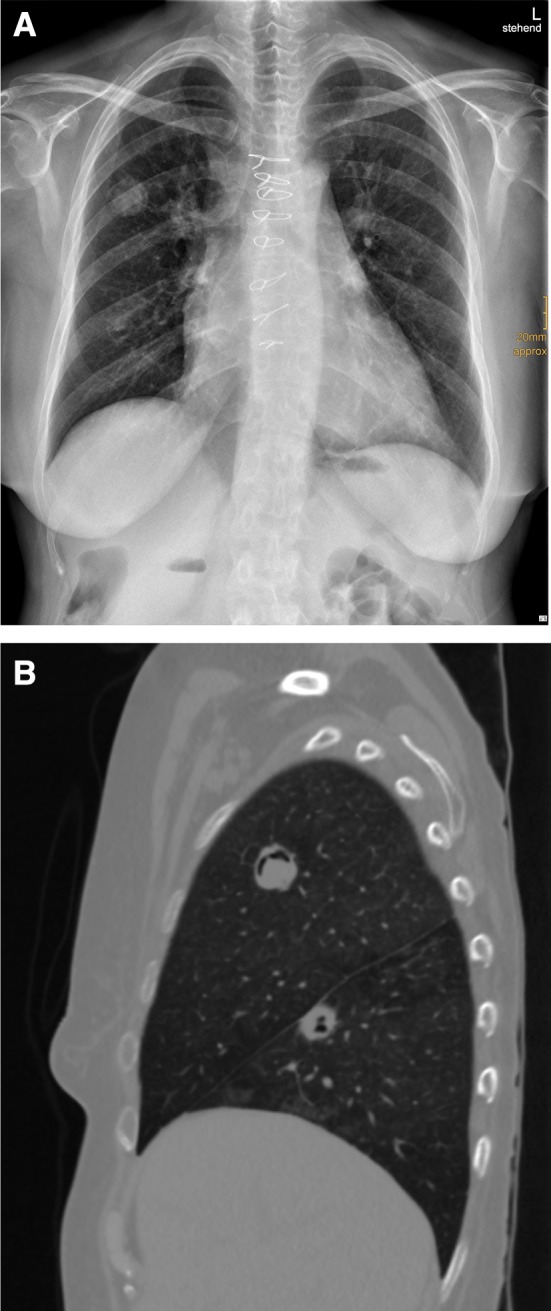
X-ray and computer tomographic images of a 52-year-old immunocompromised patient after heart transplantation suffering from pulmonary aspergillosis (*Aspergillus terreus).*
